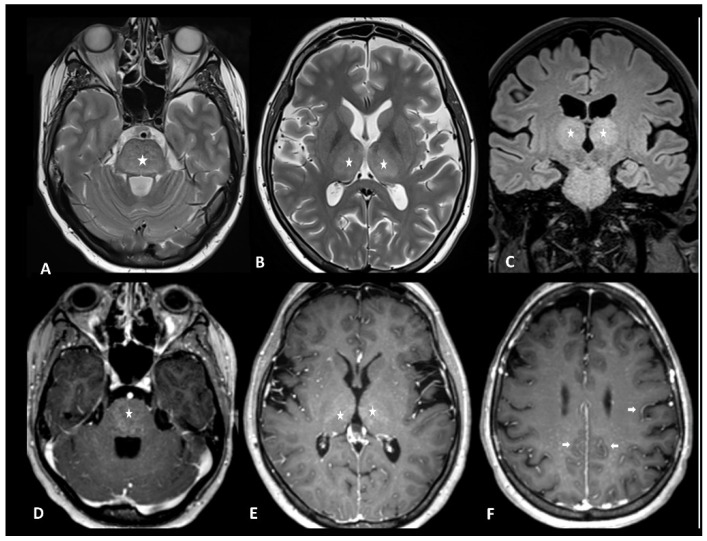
Immune effector cell-associated neurotoxicity syndrome in a 28-year-old female patient suffering from diffuse large B-cell lymphoma. The patient was admitted for CAR T infusion in the context of disease progression. Twenty-four hours after the first infusion, the patient developed fever, tachycardia, dysphagia, and dyskinesia. MRI revealed bithalamic and brain stem hyperintensities (asterisks) on T2 and FLAIR (A–C) and contrast enhancement (D,E). Diffused perivascular spaces enhancement (arrows) was also observed (F). No sign of infection was found during biological tests. Despite intravenous methylprednisolone infusion, the patient died 5 days later.
